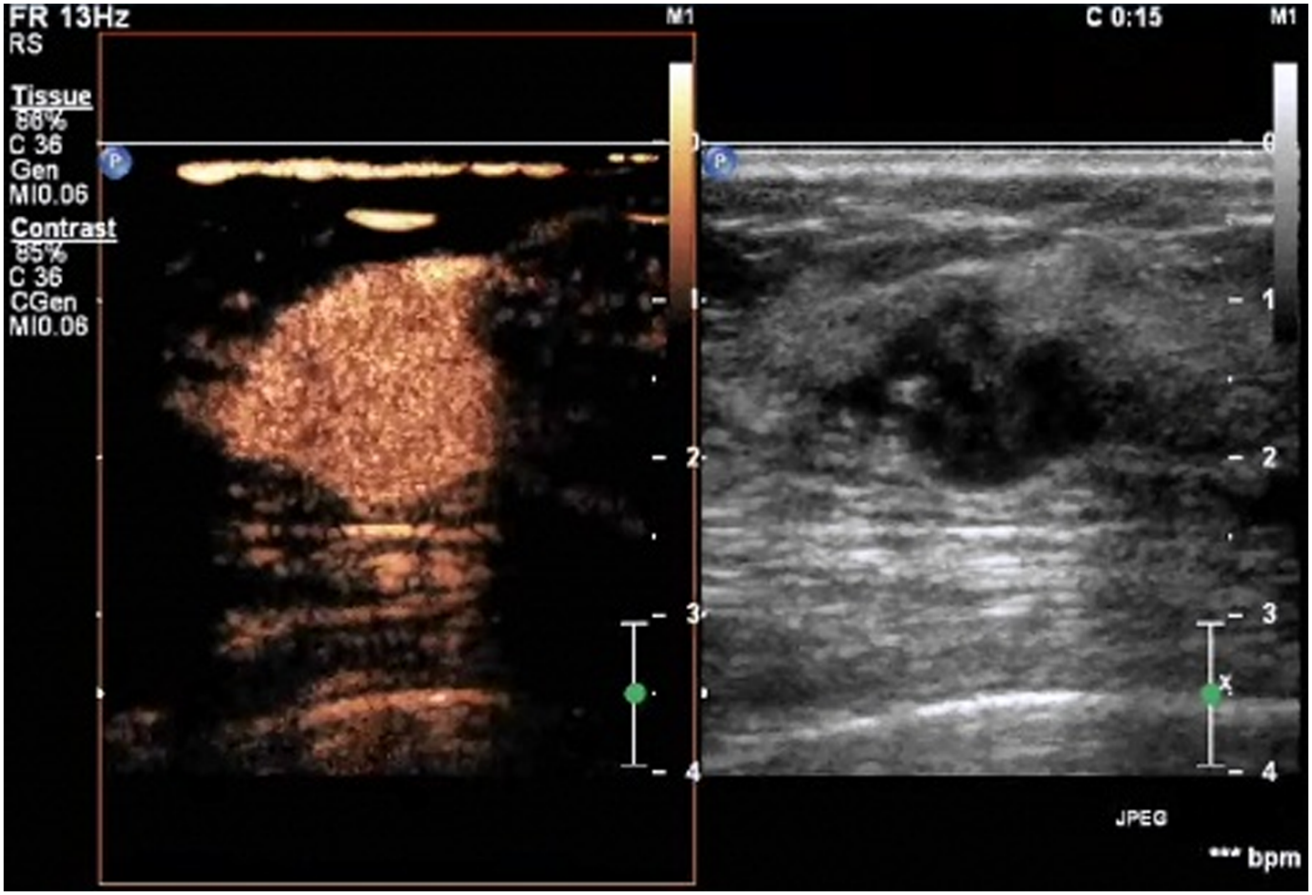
A lesion with a score of 4 by breast contrast-enhanced ultrasound. The lesion displays earlier enhancement than the surrounding tissue, usually heterogeneous. The scope of the lesion in the contrast-enhanced image is larger than in the corresponding 2D image, but the lesion still displays a clear margin, with/without a perfusion defect in the lesions and without crab claw-like enhancement.
